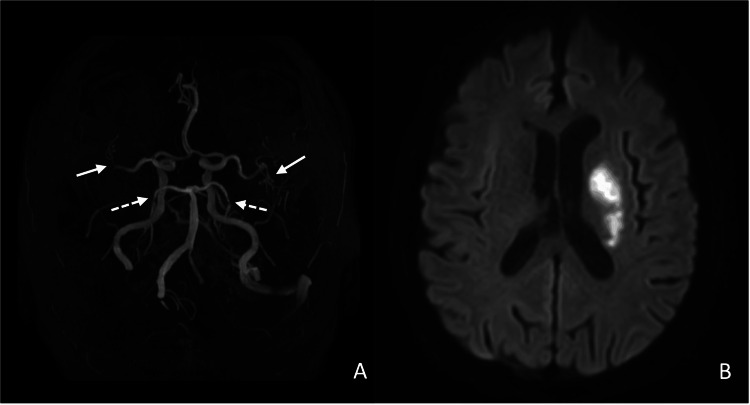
Intracranial stenoses and acute ischaemic stroke in polyarteritis nodosa (A) Magnetic resonance angiography (MRA) of the intracranial vasculature demonstrates multiple marked stenoses in the proximal segments of both the middle (full arrows) and posterior (dashed arrows) cerebral arteries. (B) Axial diffusion-weighted imaging (DWI) demonstrates an acute ischaemic lesion involving the left lentiform and caudate nuclei, as well as the corona radiata, within the vascular territory of the perforating branches of the left middle cerebral artery.
